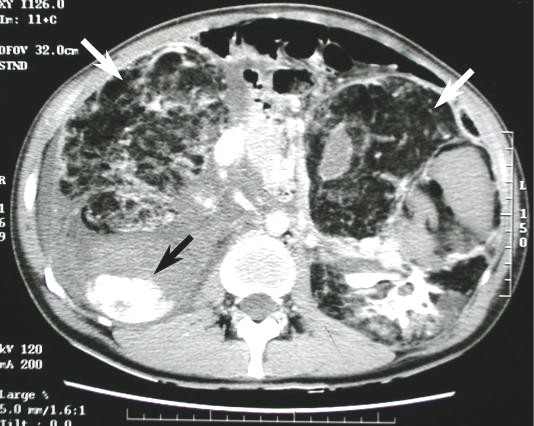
CT scan showing honeycomb kidneys (white arrows) with contrast blush inside a right retroperitoneal hematoma (black arrow).
